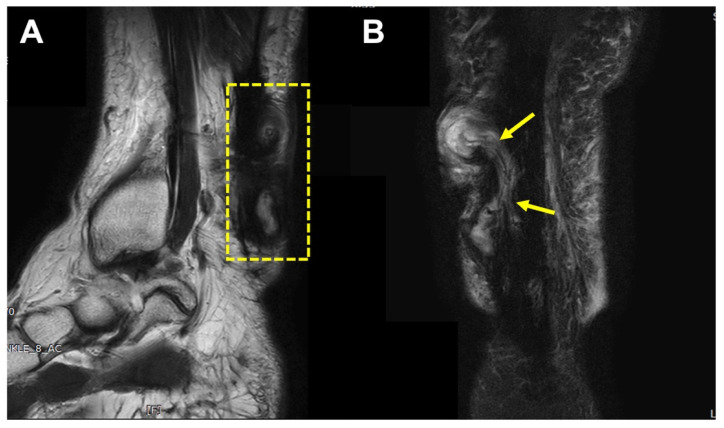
MRI findings of patient No. 1. (A) Two high-signaled, irregularly walled cystic structures (2 × 3, 2 × 2.5 cm sized) within the Achilles tendon substance (dotted box). (B) The cystic structures are communicated laterally with subcutaneous space through draining sinuses (arrows).
